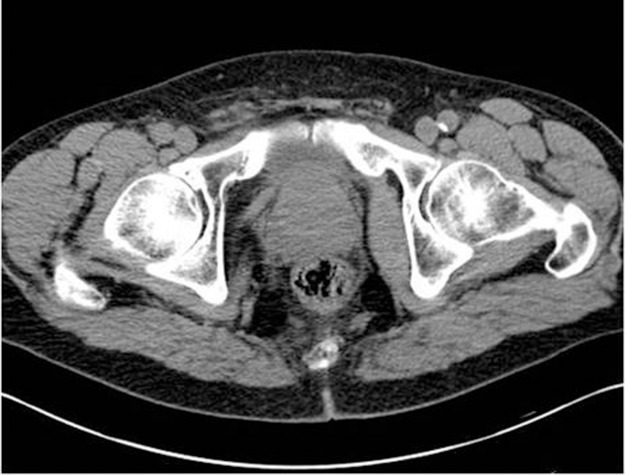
Computed tomography showing no advanced prostate disease and no metastatic bony disease
